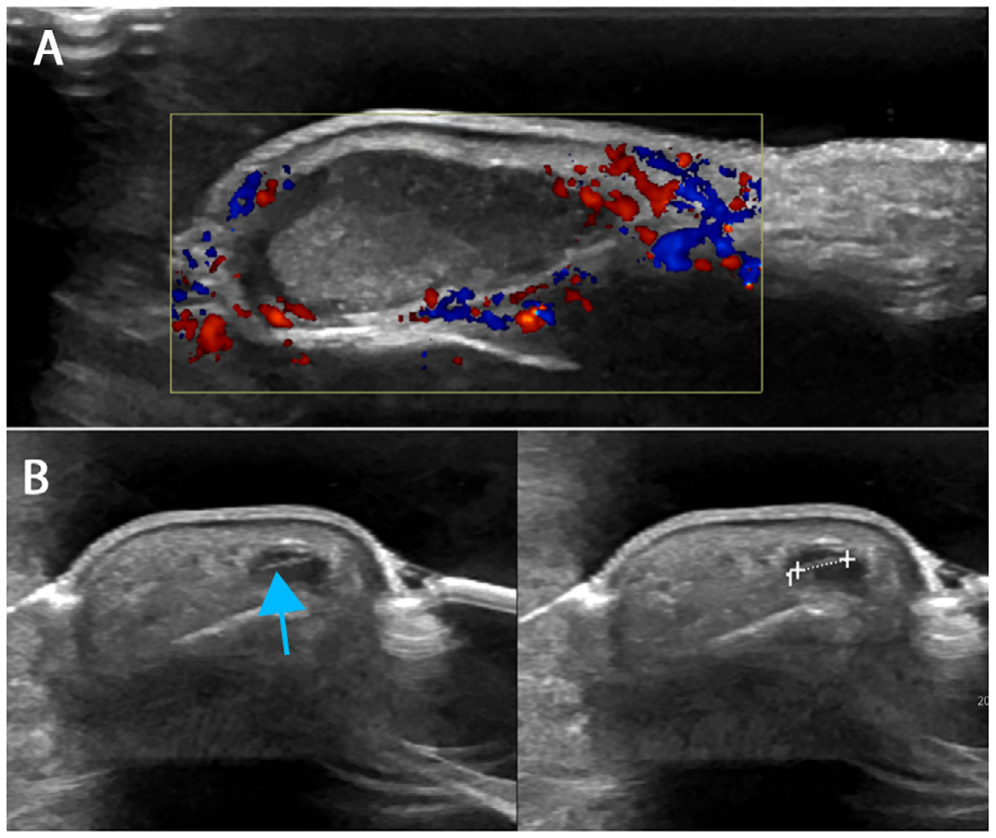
(A) Longitudinal and (B) transverse ultrasound images of the left third finger (2012) demonstrate a hypoechoic, pear-shaped subcutaneous lesion with mixed echogenicity and increased perifocal doppler activity within the surrounding soft tissues. A linear hyperechoic focus, suggestive of a retained foreign body, is identified at a depth of 5-6 mm (blue arrow).
